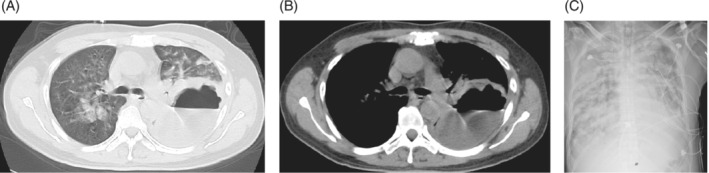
(A) The patient's chest CT lung window on admission. (B) The patient's chest CT mediastinal window on admission. (C) Chest X‐ray of the patient on admission.
